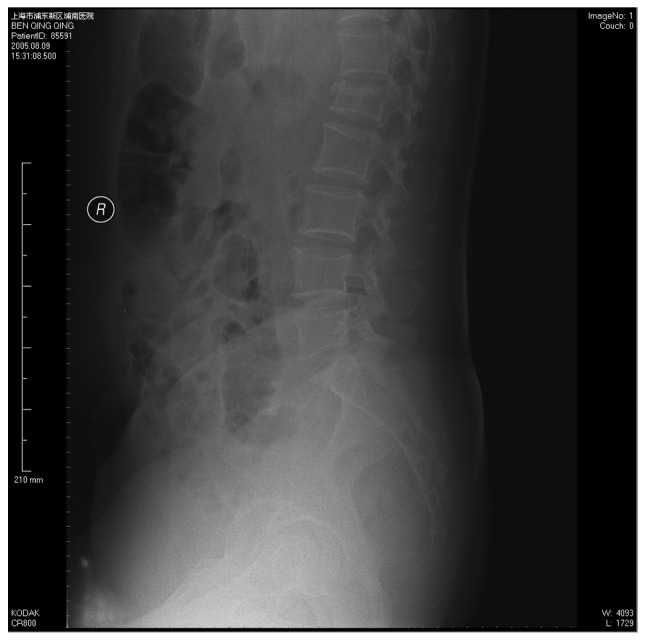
Lateral radiograph prior to dual-plane fixation of an L1 fracture.
